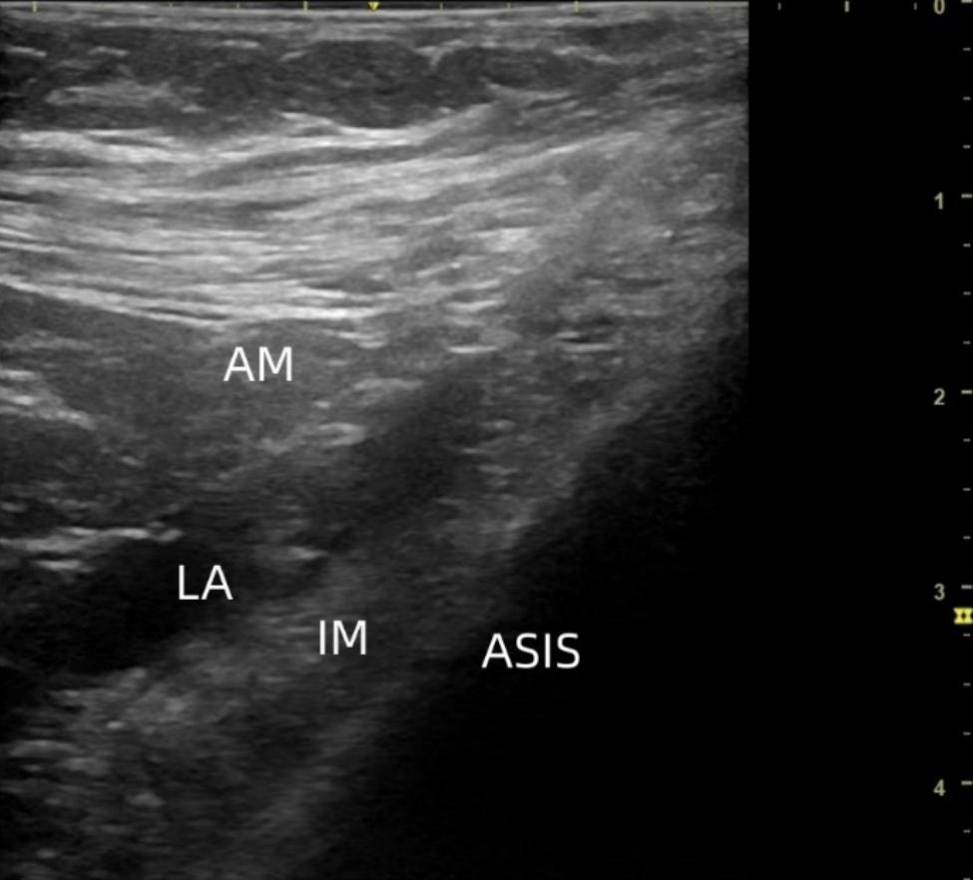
Ultrasound image of supra-inguinal fascia iliaca compartment block. ASIS Anterior superior iliac spine, IM iliacus muscle, AM abdominal muscles, LA local anesthetic
